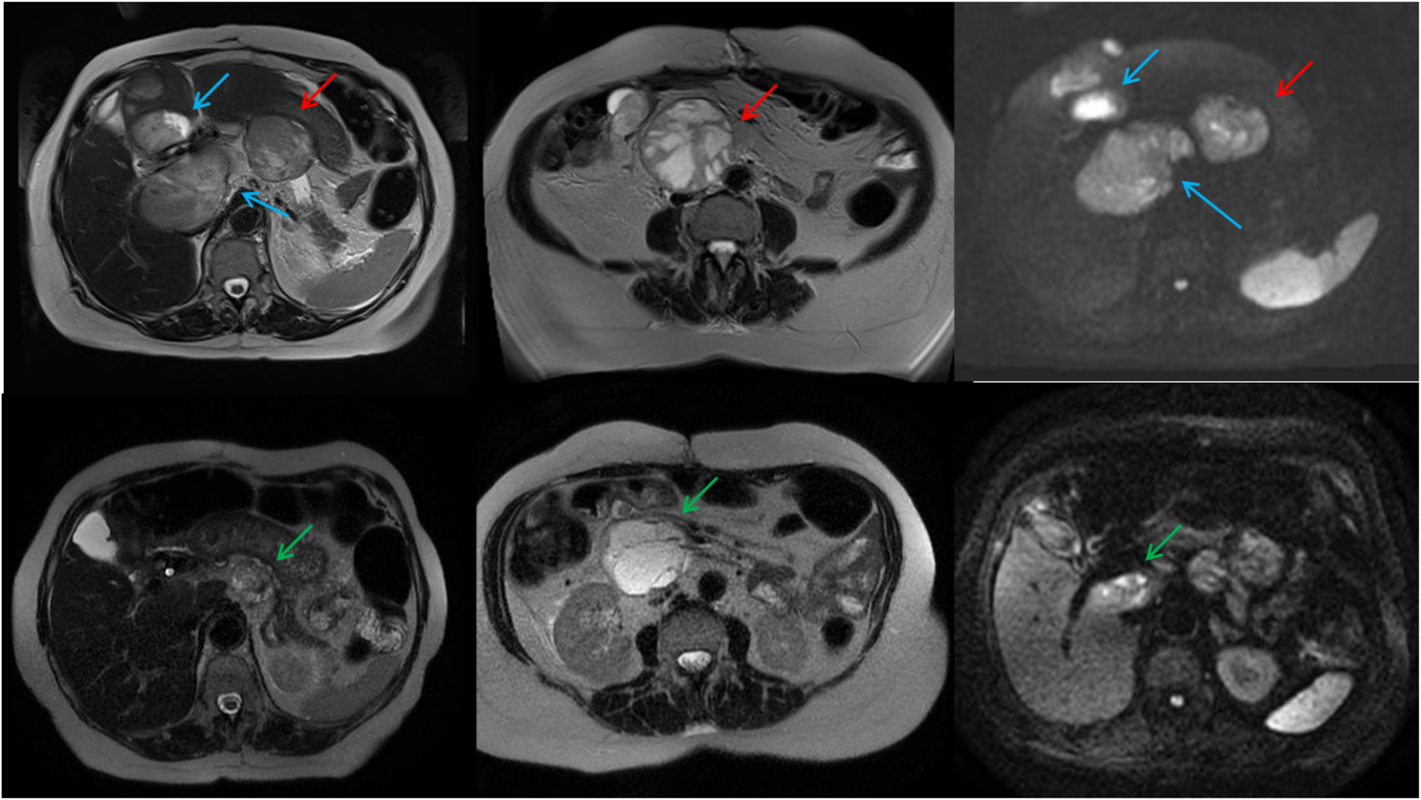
T2- and diffusion-weighted magnetic resonance imaging scans (b = 600). In the *top row*, *blue arrows* indicate hepatic lesions and *red arrows* indicate abdominal lymphadenopathy at baseline. In the *bottom row*, scans taken after metronomic chemotherapy treatment show a major reduction at the hepatic lesions (*green arrows*) as well as at the abdominal lymphadenopathy
